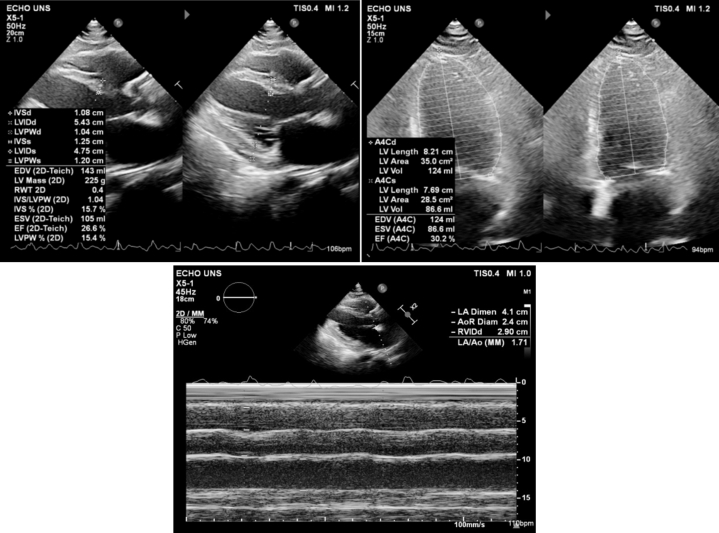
Findings on Transthoracic Echocardiography Echocardiographic assessment revealed left atrial dilatation, eccentric left ventricular hypertrophy, and segmental wall motion abnormalities. The left ventricular ejection fraction was reduced to 30.2%, and right ventricular function was impaired (TAPSE = 8 mm).
